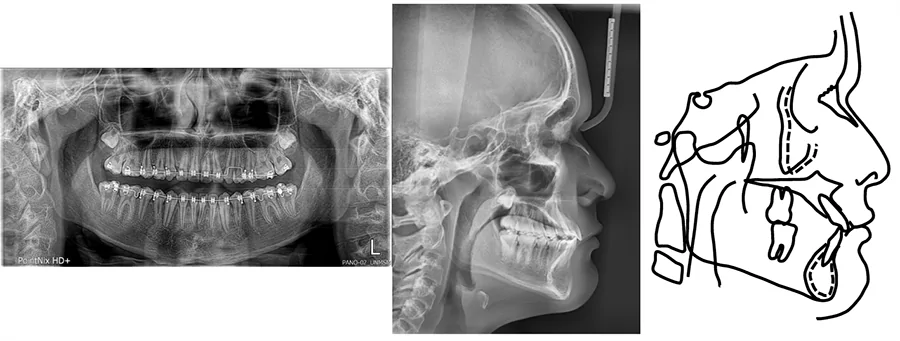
Posttreatment panoramic and lateral cephalometric radiographs, and cephalometric tracing.
